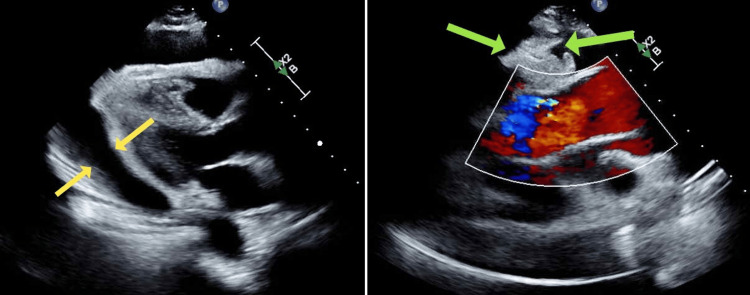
Transthoracic echocardiogram showing large, free-flowing pericardial effusion (yellow arrows) with mobile soft-tissue masses in the effusion on the visceral pericardium (green arrows).
